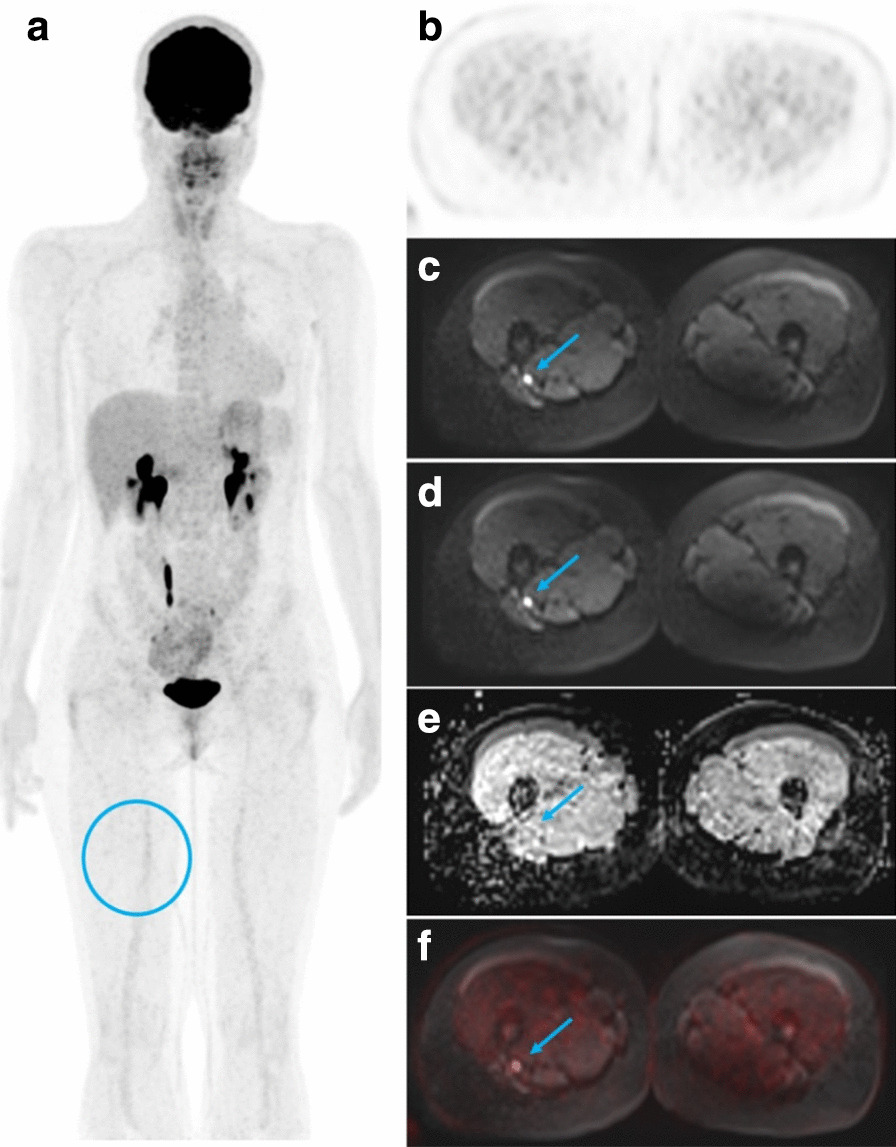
Example of a schwannoma detected by MRI but not by ^[18F]^FDG-PET. Right-sided sciatic nerve schwannoma (arrows) not visible on a coronal maximum intensity projection (MIP) ^[18F]^FDG-PET image and b axial ^[18F]^FDG-PET image (circles). Conversely, the lesion was detected on MRI as a high signal intensity focal area on c, d axial diffusion-weighted (DW) images with low and high values (50 and 800 s/mm^2^), an intermediate signal intensity on e axial apparent diffusion coefficient (ADC) image. f Axial fused ^[18F]^FDG-TEP and *b* = 800 s/mm^2^ DW image
